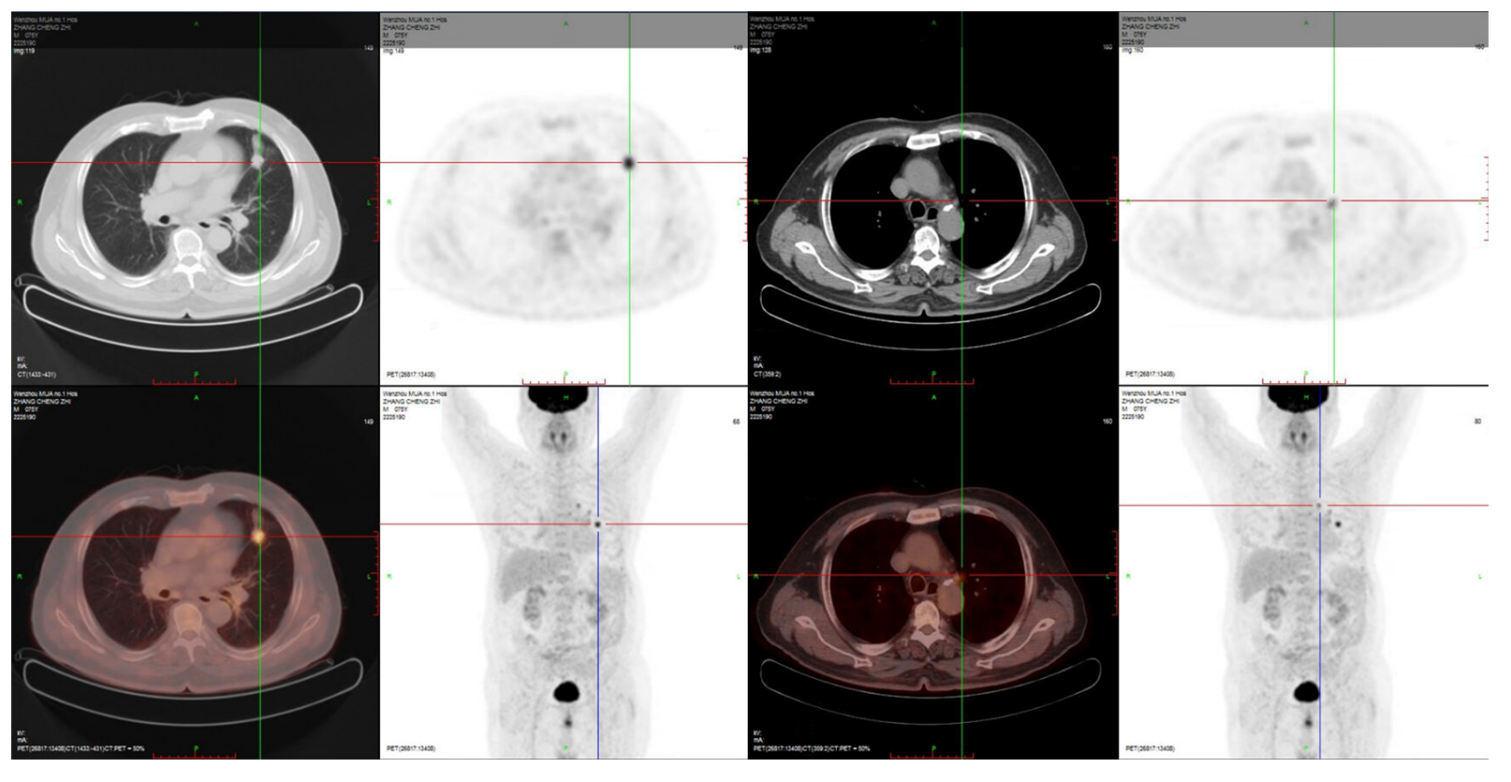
PET-CT on 4 November 2020.
